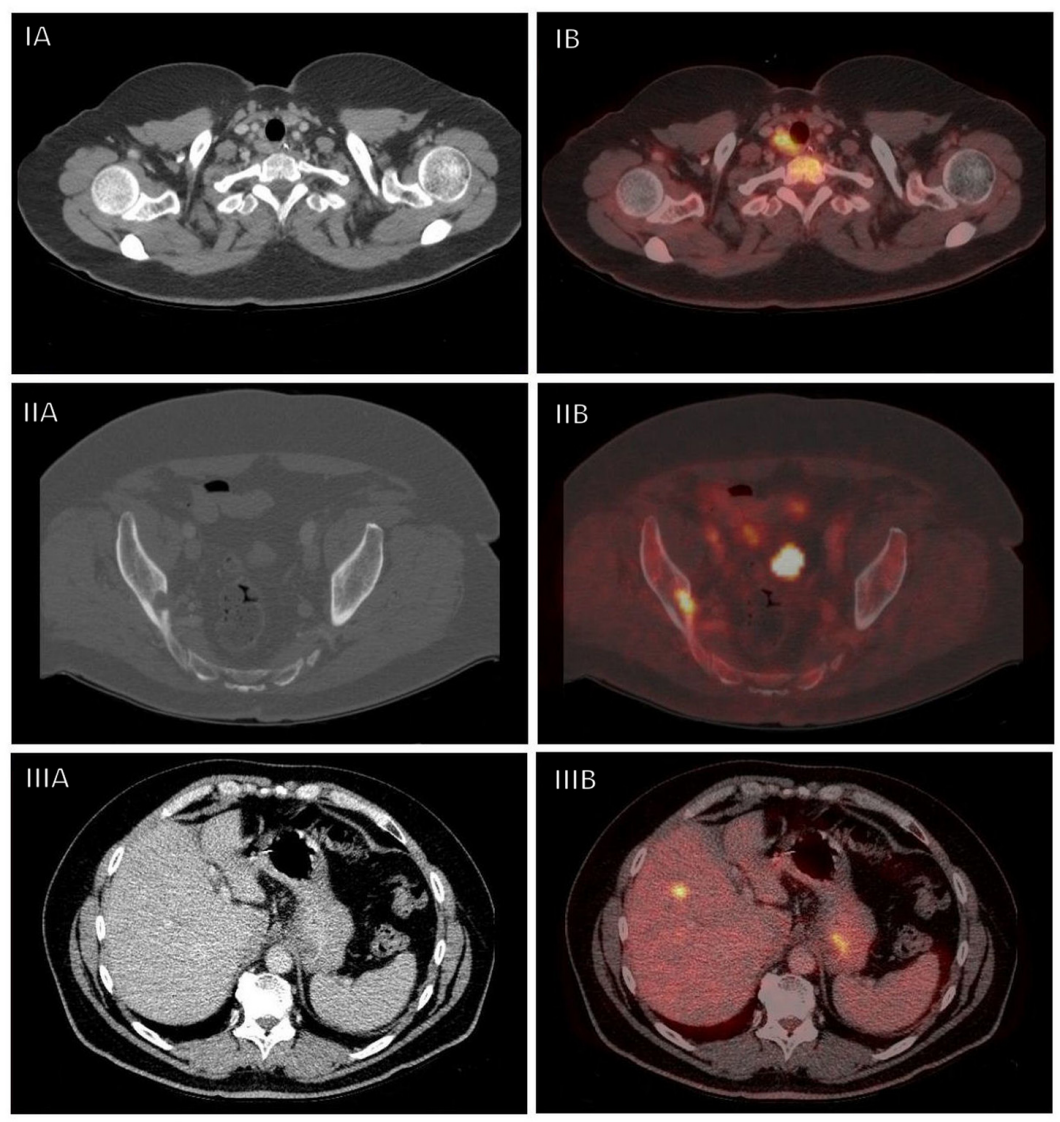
Examples of metastatic disease detected with restaging PET-CT. Examples of interval metastases on restaging PET-CT located in a supraclavicular lymph node (I), the right iliac body (II) and the liver (III). Panel A and B represent axial CT images before and after fusion with PET, respectively.
